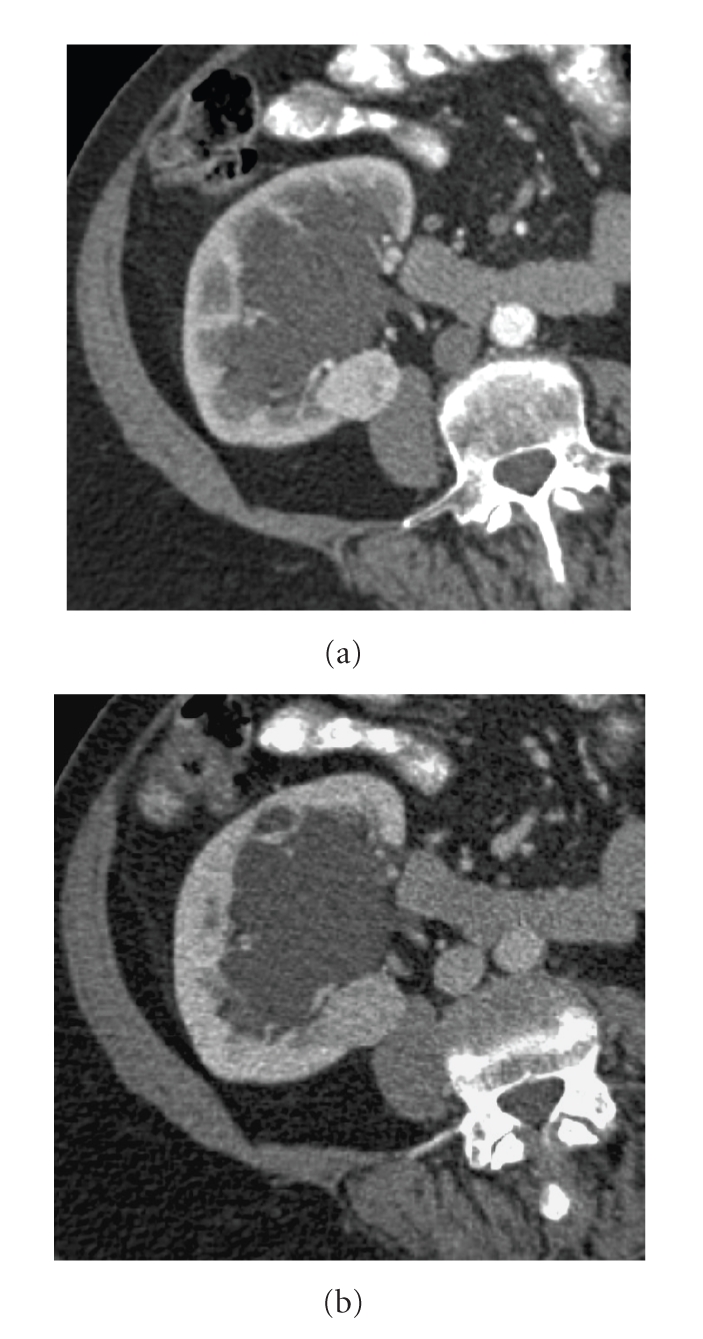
Small hyper vascular renal cell carcinoma. (a) Contrast-enhanced CT shows
